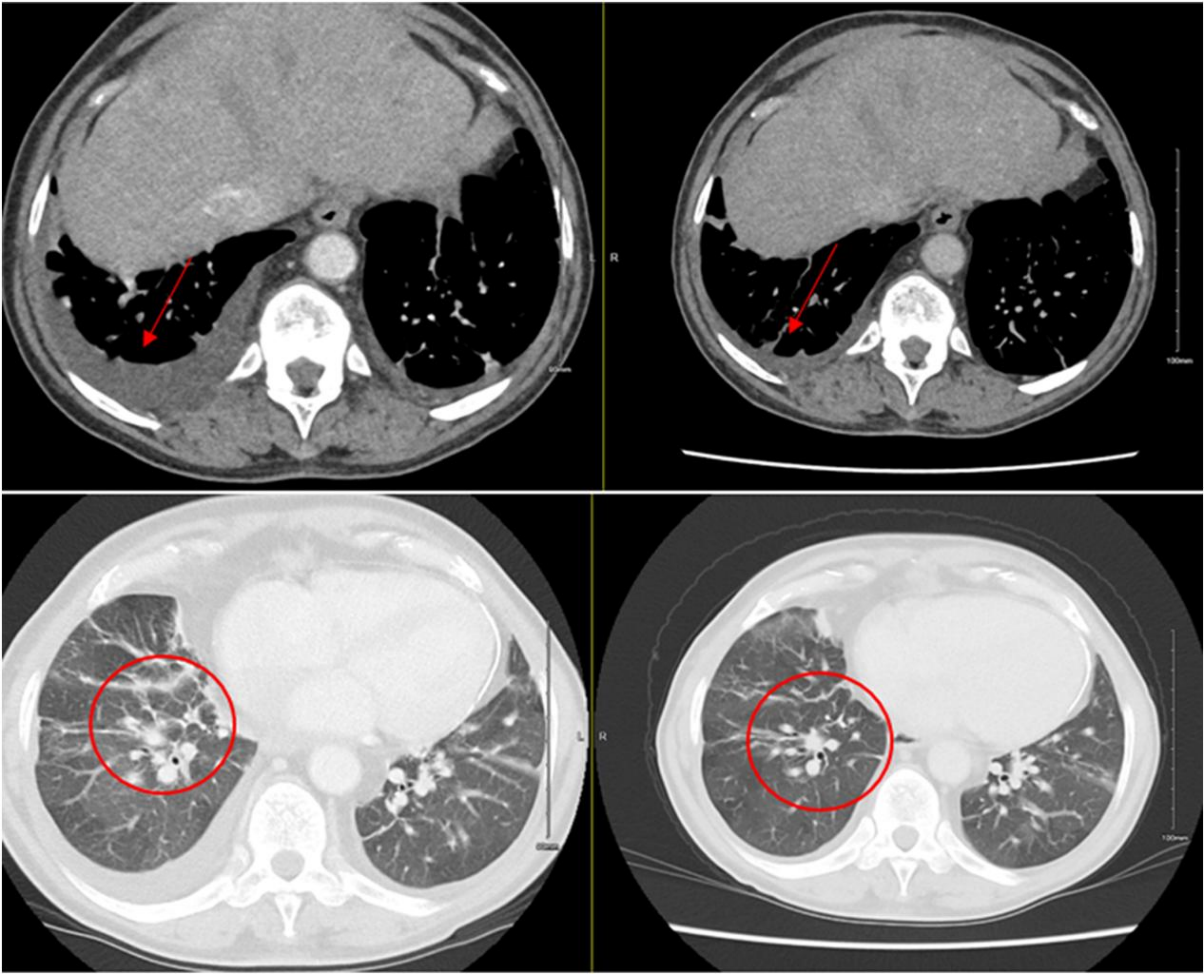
Comparison of pleural effusions and peri-bronchial thickening between initial and subsequent computed tomography thorax, indicating worsening heart failure.
